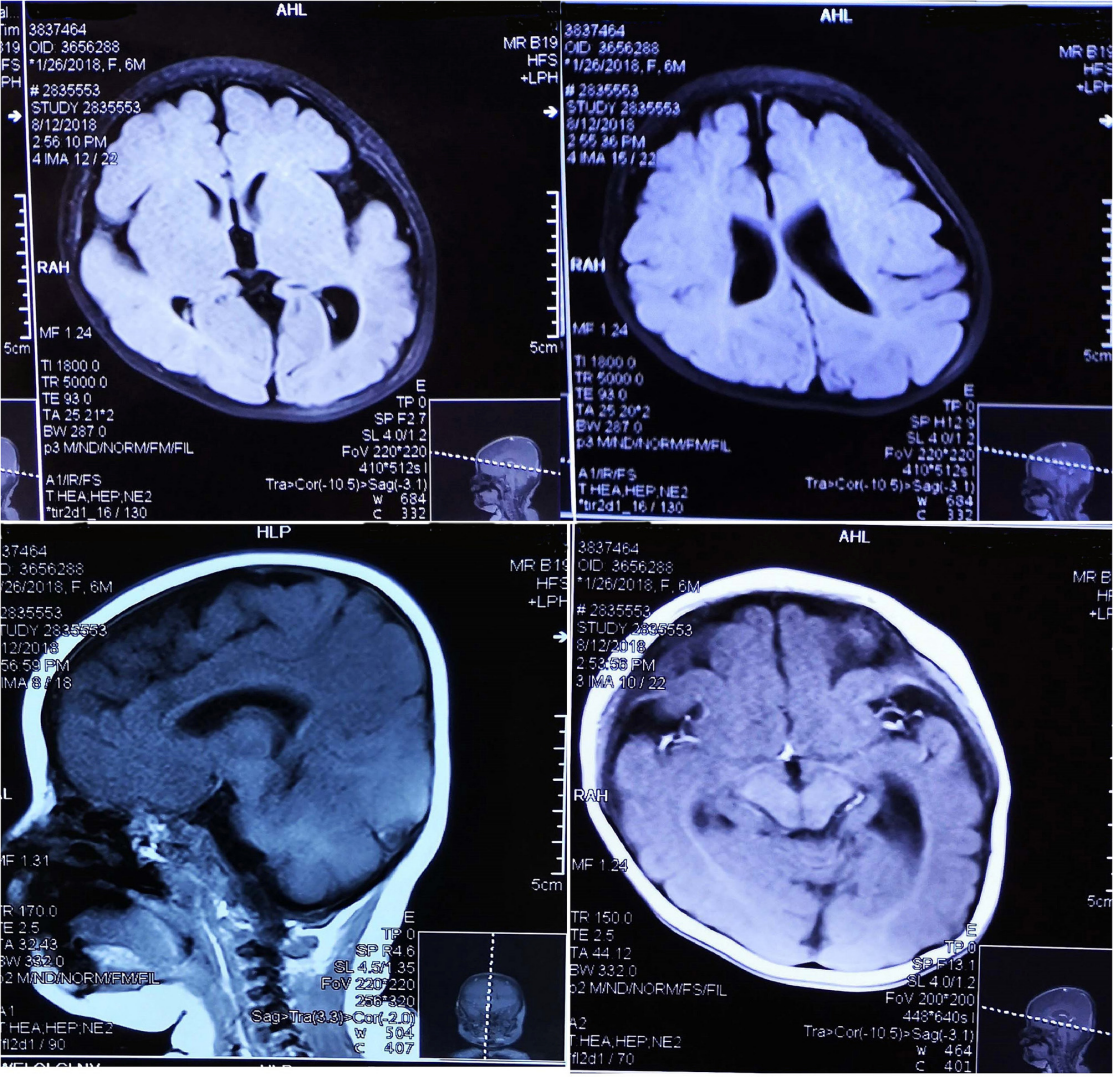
The brain MRI of patient 3 showed MCD.
